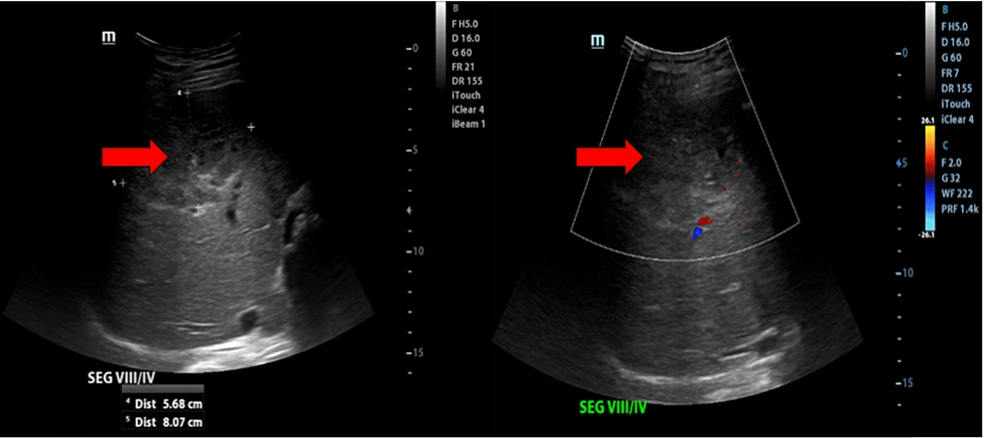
Ultrasound abdomen showing non-liquefied liver abscess at segment IV/VIII measuring 5.7cm x 8.1cm (AP x W).
